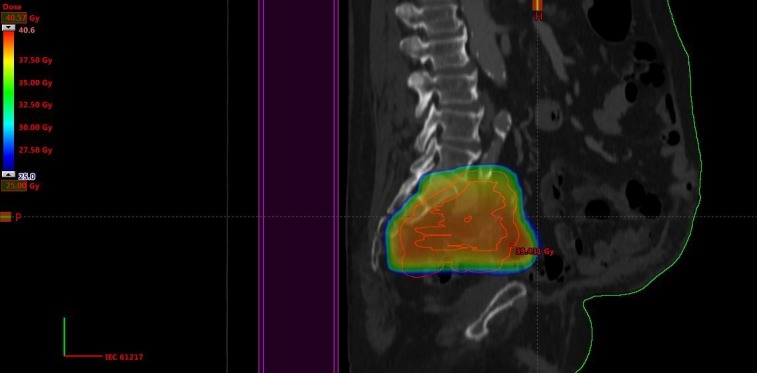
Sagittal computed tomography (CT) scan and dose distribution Doses above 25 Gy are displayed, maximum dose 41 Gy.
